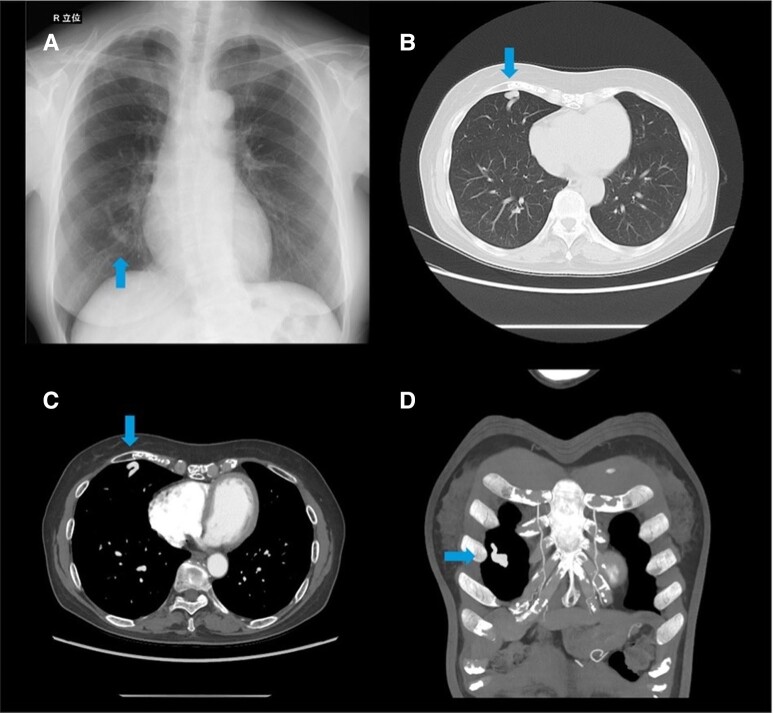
Pulmonary arteriovenous malformation. (*A*) Chest X-ray: increased vascular markings in the right lower lung (arrow). (*B–D*) Chest CT: pulmonary arteriovenous malformation (blue arrow) (*B* non-contrast; *C*, *D* contrast enhanced).
